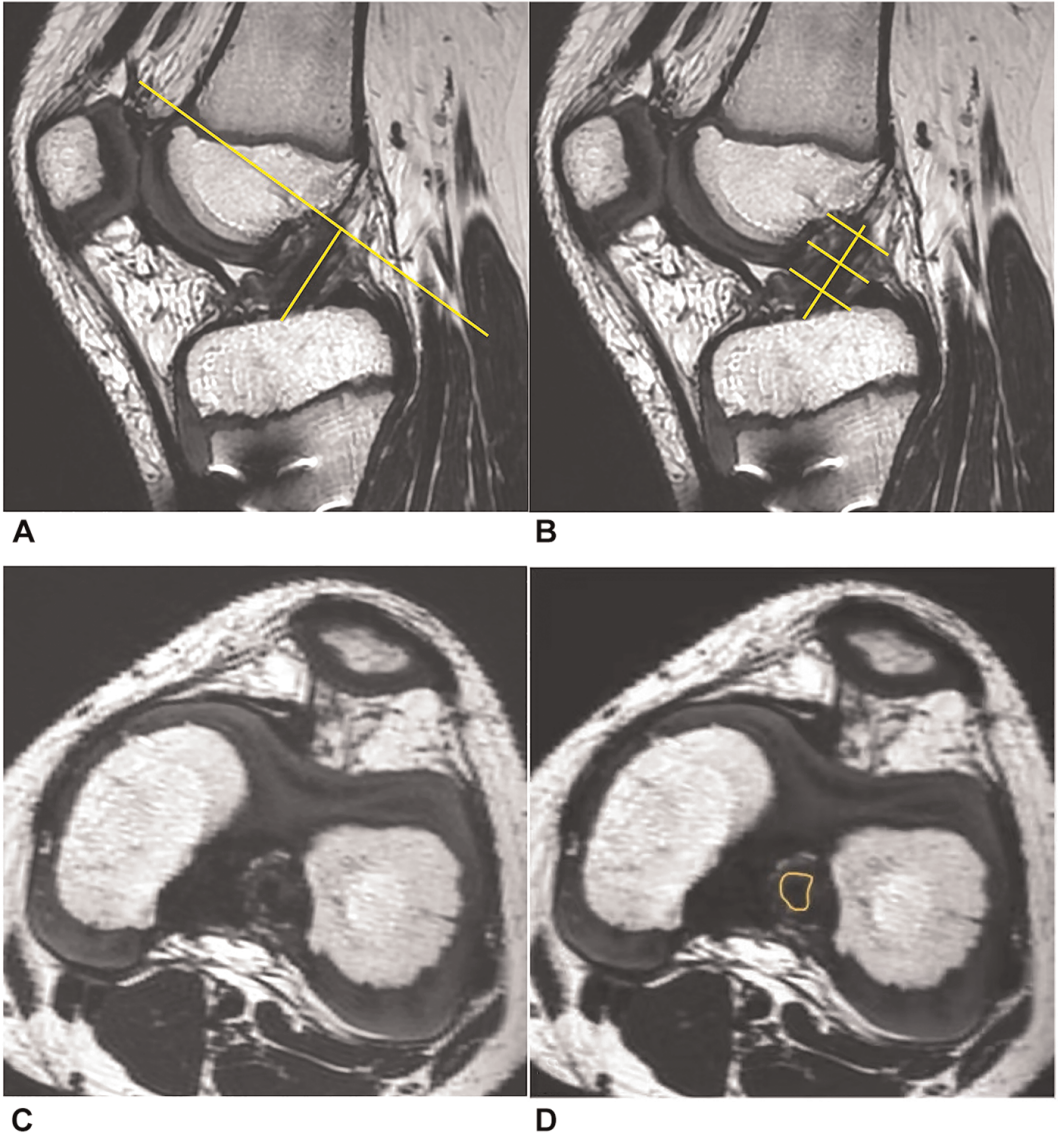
3D-MRI assessment of the ACL. (A) A reference line across the center of the entire ACL graft and a perpendicular plane. (B) The 2nd, 3rd, and 4th slices represented three evenly spaced cross-sectional slices (at 25%, 50%, and 75% of the ligament's overall length from the femoral attachment to the tibial attachment). (C) Cross-section of ACL graft at the mid-point location. (D) Outline of the cross-sectional area.
